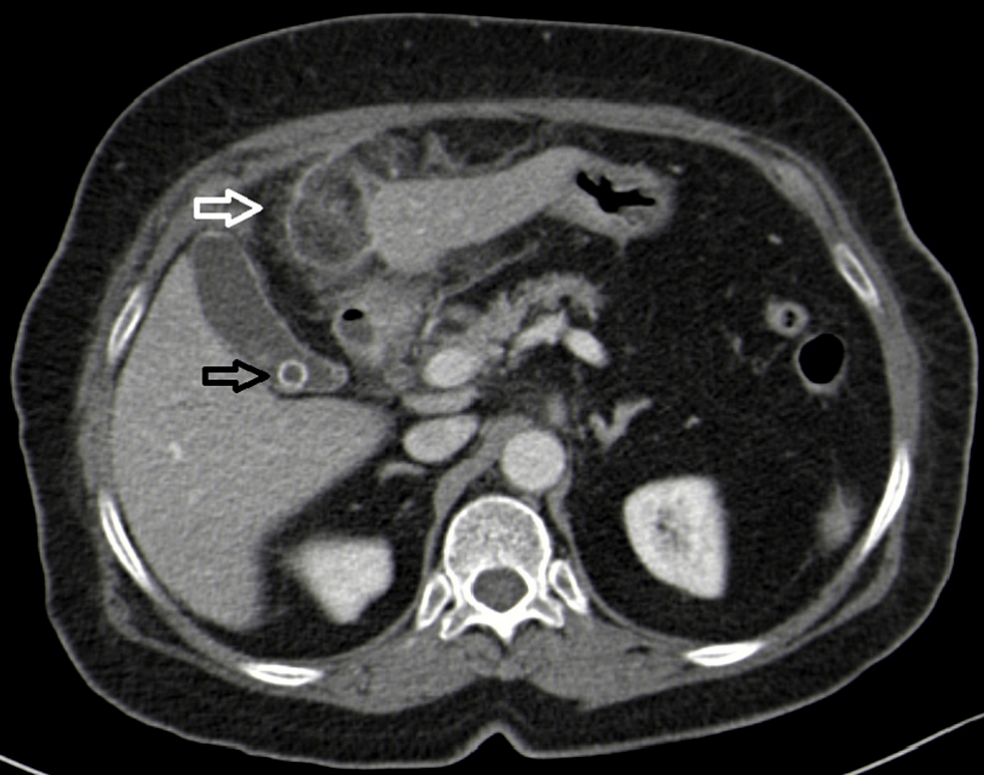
Computed tomography axial plane showing heterogeneous increase density of fat and hyperattenuating rim sign adjacent to falciform ligament, compatible with falciform ligament appendagitis (white arrow). Vesicular lithiasis with normal gallbladder appearance can also be seen (black arrow).
